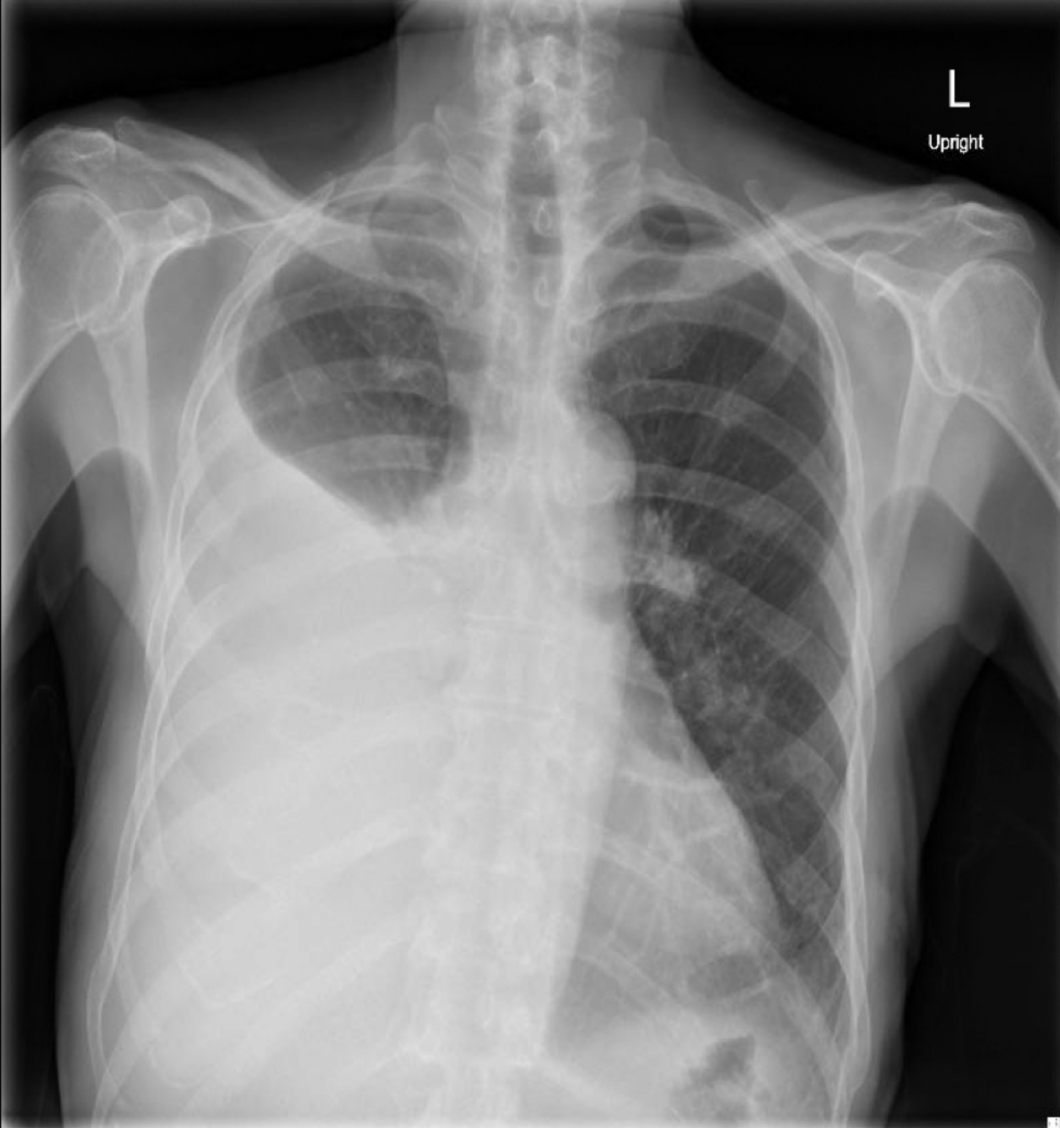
Chest radiograph (anterior-posterior projection) showing large right-sided pleural effusion
